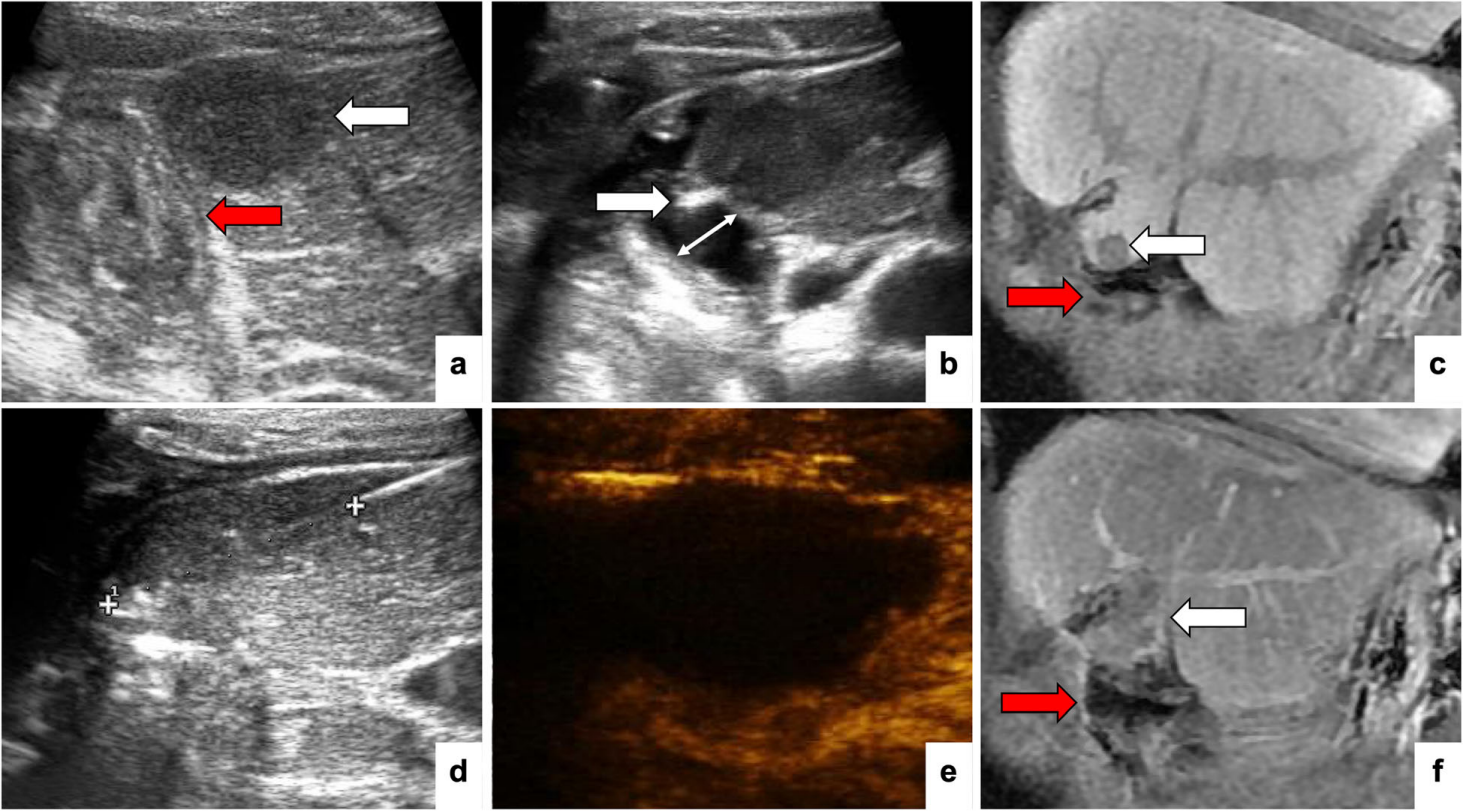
A patient with a history of hepatectomy and cholecystectomy. The index tumor was in segment 4 and ablated with RFA. Ultrasound (a) and MR (c) images show that the distance between the index tumor (white arrow) and intestine (red arrow) was < 5 mm. b A PTC needle is inserted with the tip (arrow) in the gap between the tumor and the intestine. Perfusion of normal saline established a local thermal barrier (double arrow line). d The RFA zone was about 3 cm × 2 cm and was measured 3 cm along the needle tract to control the size. After ablation, CEUS (e) and CEMR (f) show that the tumor was completely ablated
